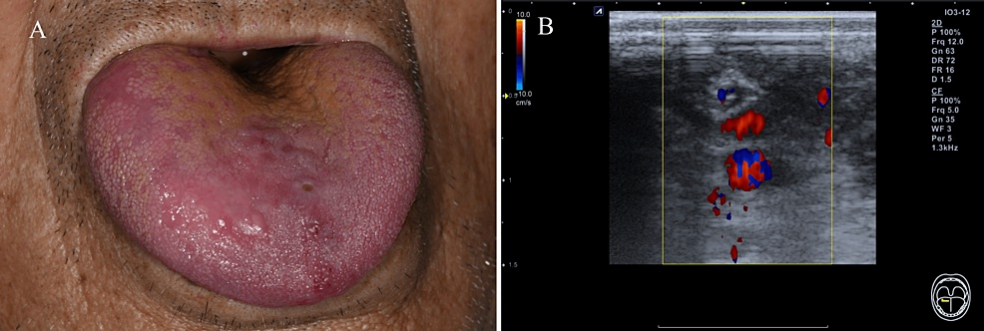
Vascular lesion after sclerotherapy (A) seven-month follow-up appearance (B) four-month follow-up ultrasonography doppler image, which shows remarkably decreased size and flow of the lesion.
